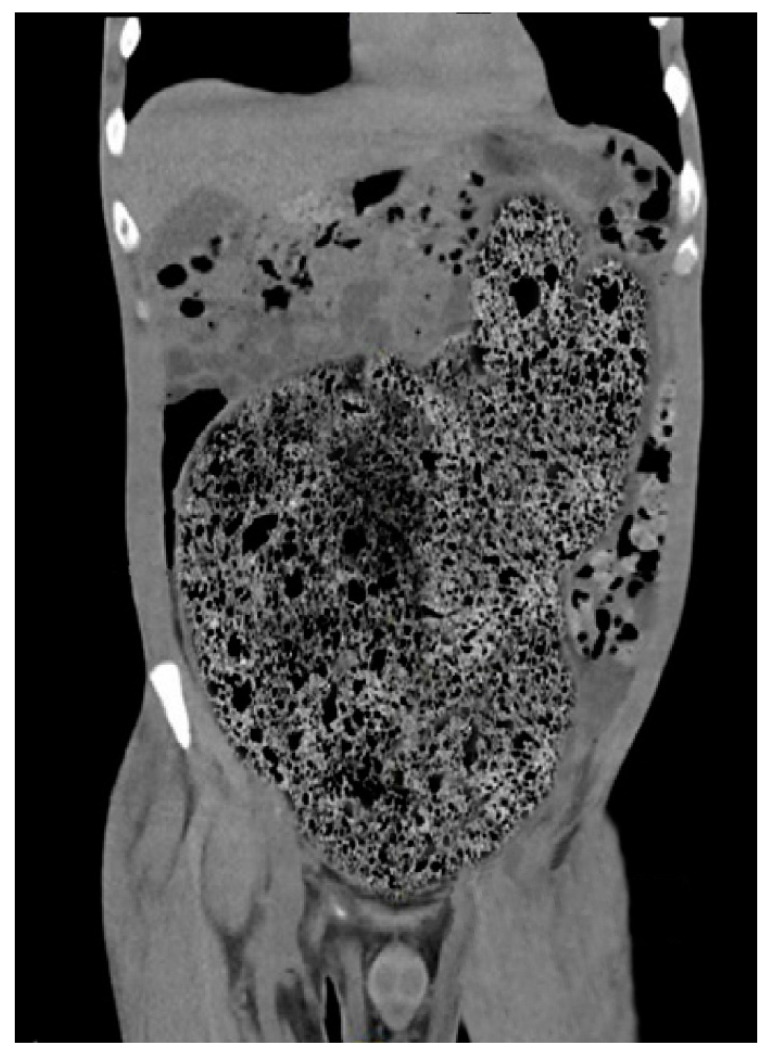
CT scan with idiopathic megarectum and megacolon.
